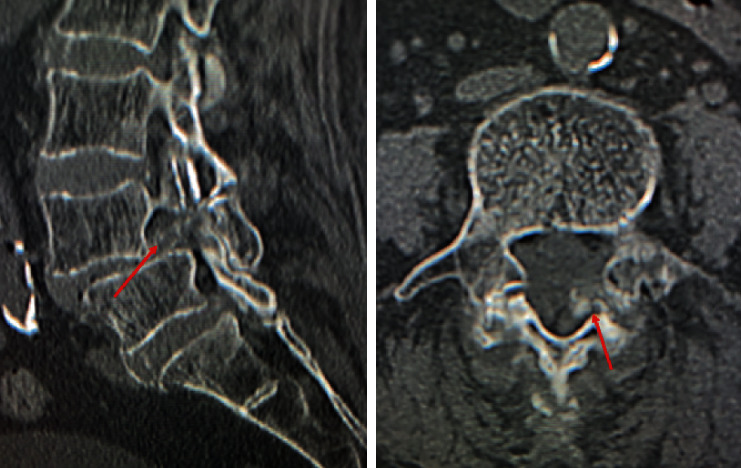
A nodular calcification mass was observed from the location of left L4 spondylolysis to the intraspinal canal in plain CT images.
